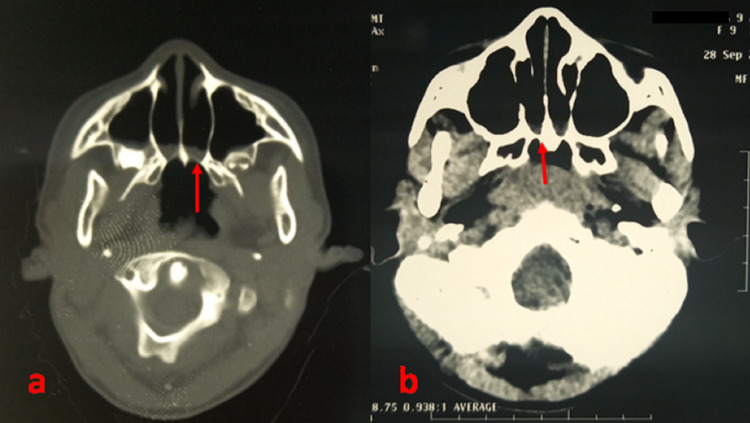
CT scan of an osteomembranous choanal atresia (red arrow) in axial sections bone window (a) and parenchymal window (b).
